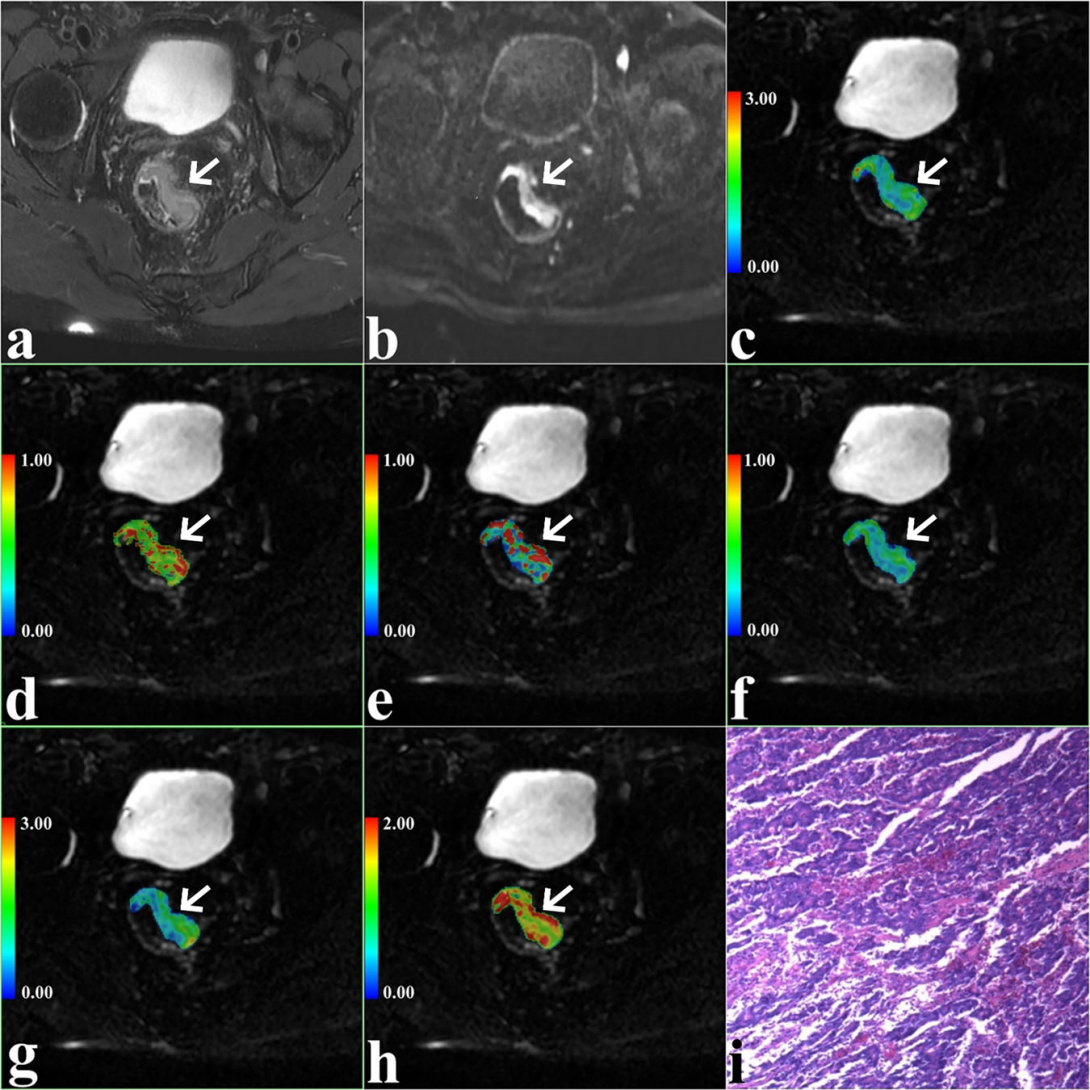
A 67-year-old man with LNM-positive rectal cancer in the left wall of rectum (arrow, pseudo colored region). T2-weighted image showed a slightly hyperintensity mass (a) with restricted diffusion on DWI (b) ADC map (c). d–h f_1_, f_2_, f_3_, D_app_, and K_app_ maps of the same slice as in a–c. i Pathological image (original magnification, ×100)
